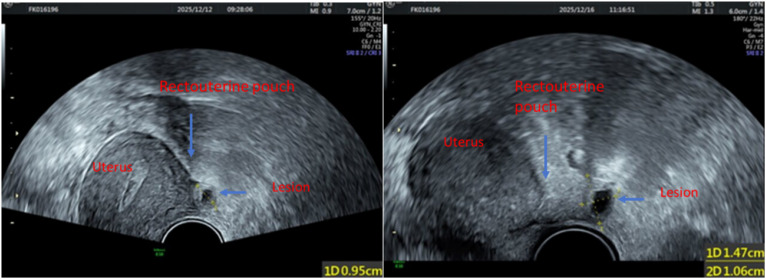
Transvaginal ultrasonography obtained at a 4-day interval (left: initial scan; right: follow-up scan), demonstrating heterogeneous endometrial echoes and a lesion located posterior to the uterus (transverse view). Key anatomical structures are labeled, including the uterus, the lesion, and the rectouterine pouch (pouch of Douglas).
